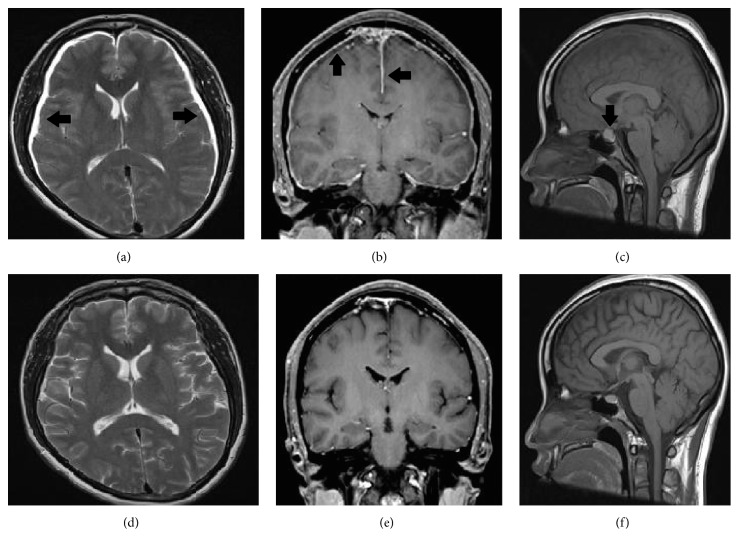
(a) Axial T2 weighted sequence demonstrates bilateral CSF-intensity subdural hygromas (arrows). (b) Coronal T1 weighted gadolinium enhanced sequence demonstrates pachymeningeal thickening and enhancement (arrows). (c) Sagittal T1 weighted sequence demonstrates pituitary gland enlargement. (d)–(f) Posttreatment MRI examination demonstrates complete radiological resolution.
